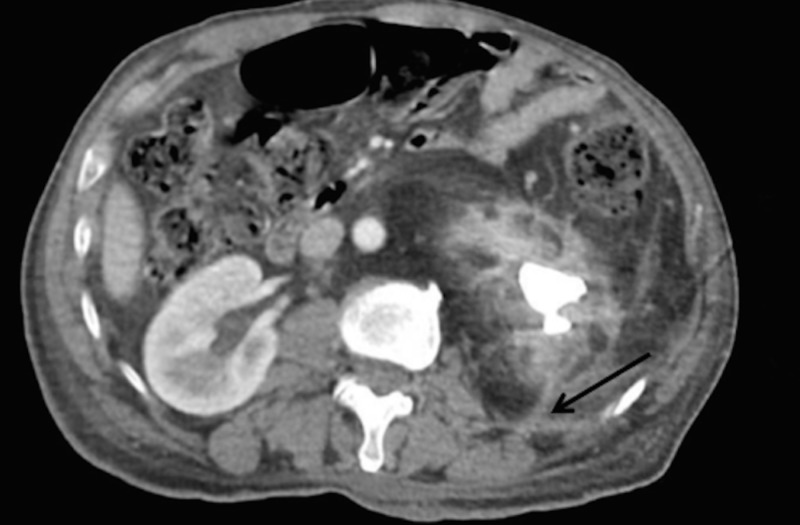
CT scan clearly delineating the formation of a nephrocutaneous fistulous tract
